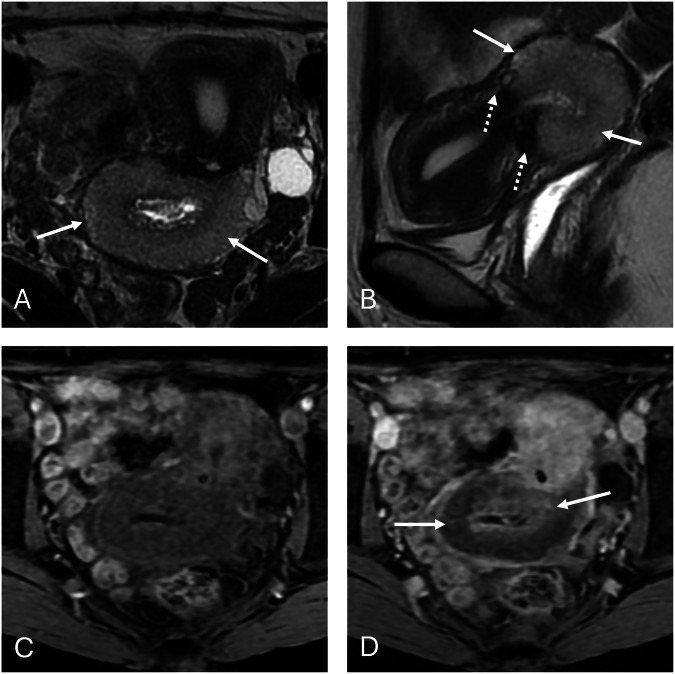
Cervical gynaecological venous malformation (GVM) in a 48-year-old patient, detected on clinical examination as cervical enlargement, raising suspicion of neoplasm. A Axial and B sagittal T2-W MR images show a well-defined, circumferential T2-hyperintense thickening of the cervix with a subtle sponge-like appearance (arrows), with no infiltrative pattern. Note the absence of flow-voids and the sharp delineation (dotted arrows) from the uterine isthmus and body, which appear normal. C Axial dynamic post-contrast T1-W fat-suppressed MR images at the arterial and D at the venous phase show a slow, progressive enhancement of the lesion (arrows), consistent with slow venous flow, without any arterial component
